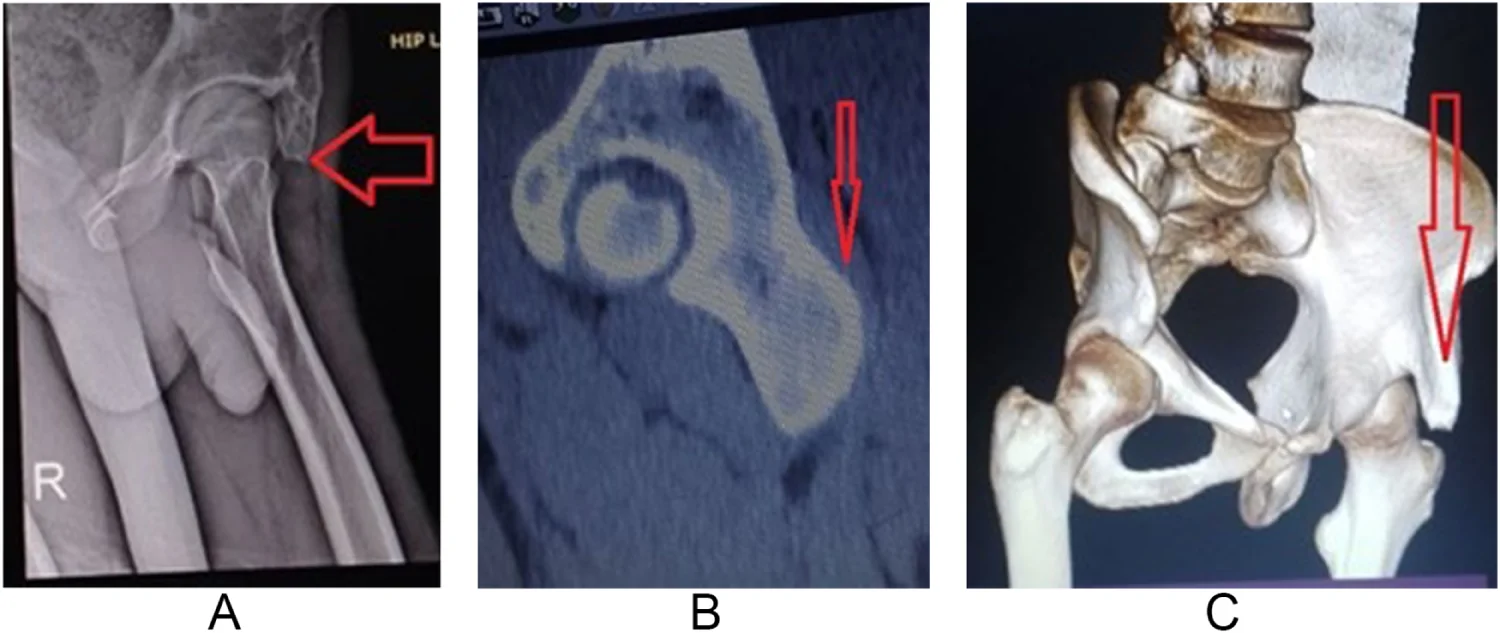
(A) Plain radiograph (anteroposterior view) showing bony exostosis (arrow) from the anterior aspect of the iliac bone. The lesion demonstrates continuity with the underlying cortex. (B, C) CT scans showing a sessile osteochondroma arising from the left anterior inferior iliac spine. The lesions (arrows) show continuity of cortical and medullary bone with the native iliac bone, characteristic of an osteochondroma.
